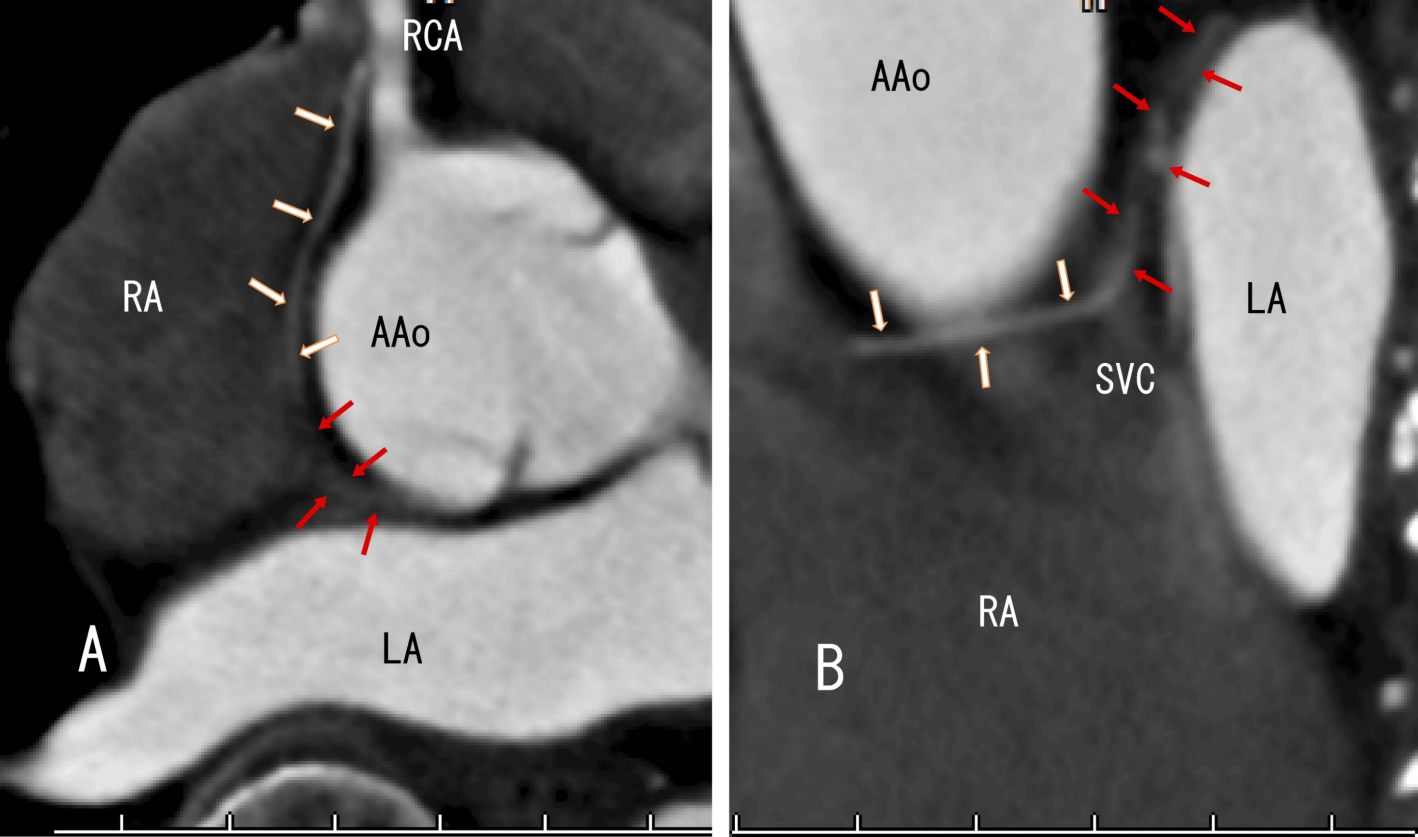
Enhanced CT images showing the AAo and LA. Enhanced CT (A: axial, B: sagittal) revealed an atypical RCA (white arrows) and continuous vague whitish areas (red arrows), suggesting an arterial blood supply. CT: computed tomography; AAo: ascending aorta; LA: left atrium; RCA: right coronary artery; RA: right atrium; SVC: superior vena cava
